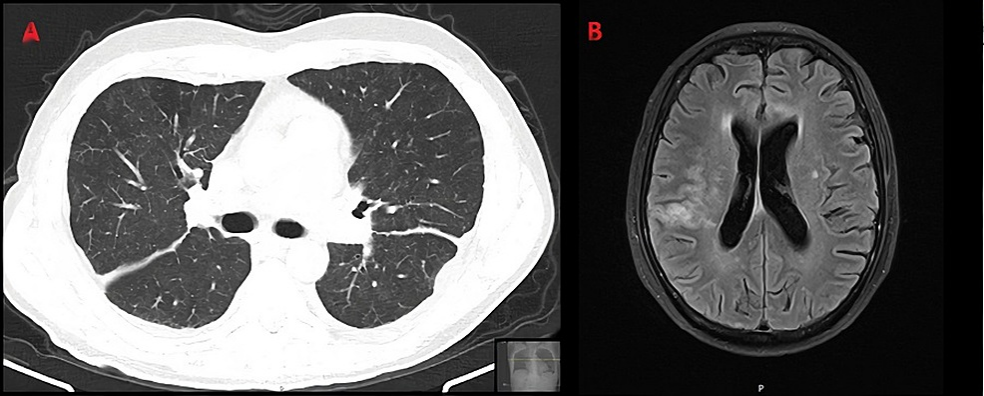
Repeat thoracic CT scan (A) and cerebral MRI (B) showing favorable evolution of the TB lesions. CT: computed tomography; MRI: magnetic resonance imaging; TB: tuberculosis
